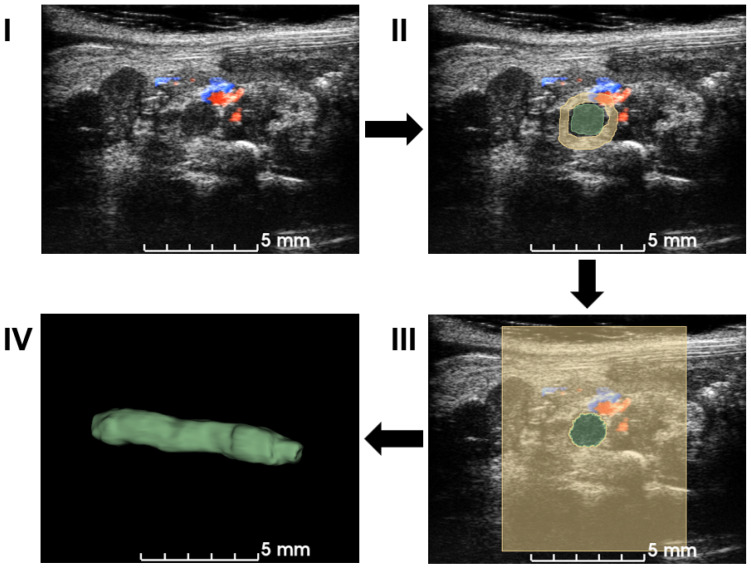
Workflow of the semiautomatic 3D segmentation. First, ultrasound volume is loaded into 3D slicer (I). Manual segmentation is performed on multiple slices and angles based on B-mode signal density and Doppler blood flow signal in (II). A seeding algorithm is run and the results are shown in a 2D view (III). Modifications are applied to extract a 3D volume of a blood clot in (IV). After the segmentation, the volume of the blood clot is calculated for quantification.
